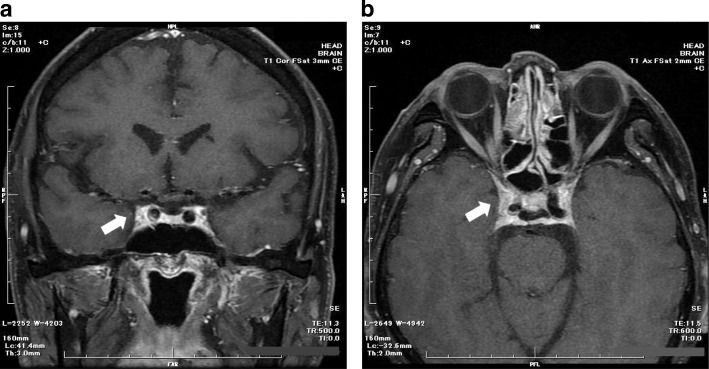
a Coronal T1 scan of brain MRI with gadolinium. b Axial T1 scan of orbital MRI with gadolinium. Both images show the lesion expanding from the right cavernous sinus to the orbital apex (arrows)
